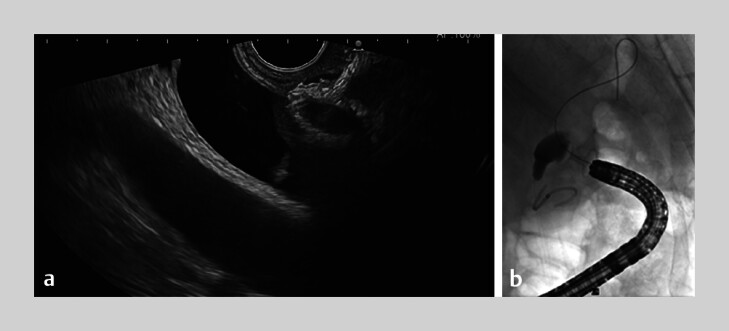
Images during endoscopic ultrasound-guided choledochoduodenostomy (EUS-CDS) showing:
